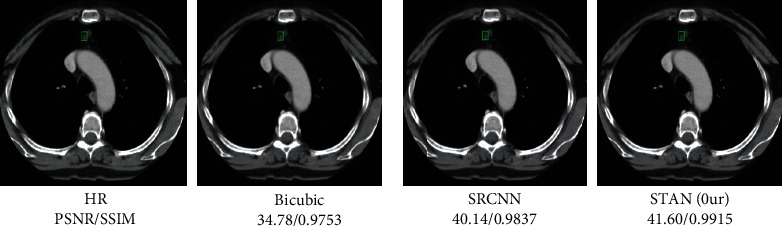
Medical CT image testing using different algorithms on the DeepLesion dataset.
